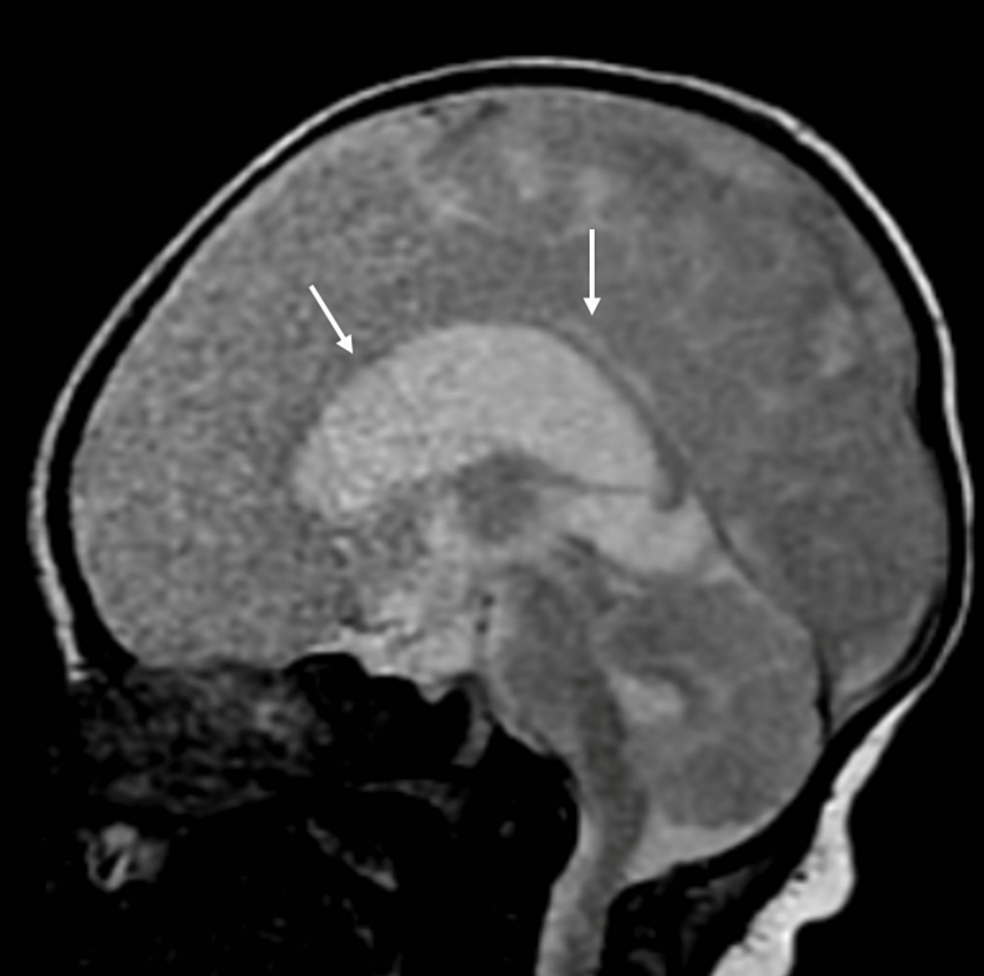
Sagittal view MRI T2WI sagittal view shows hypogenesis of the corpus callosum T2WI: T2 weighted image
